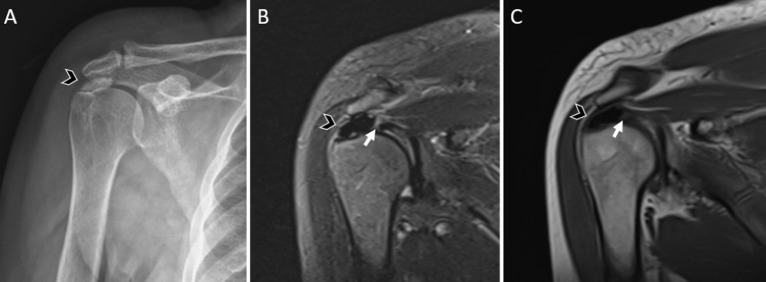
Calcific tendinitis accompanied by a tear in the same tendon and at the same anatomical location. Right shoulder images of a 60-year-old male patient.(A)Plain radiograph of the right shoulder demonstrates an approximately 17 mm calcific radiopaque focus at the supraspinatus tendon insertion (arrowhead).(B)Sagittal proton density–weighted and(C)coronal T1-weighted magnetic resonance imaging images show a low-signal-intensity calcific focus at the same location (arrowhead), together with a full-thickness tear of the supraspinatus tendon at the same anatomical level (arrow).
